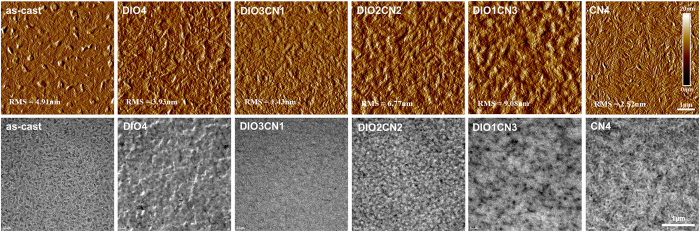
Upper panel: AFM phase images; lower panel: TEM images. In these panels, the results for the PBDTTT-CT:P(NDI2OD-T2) blend films as a function of binary additive ratios are presented.
